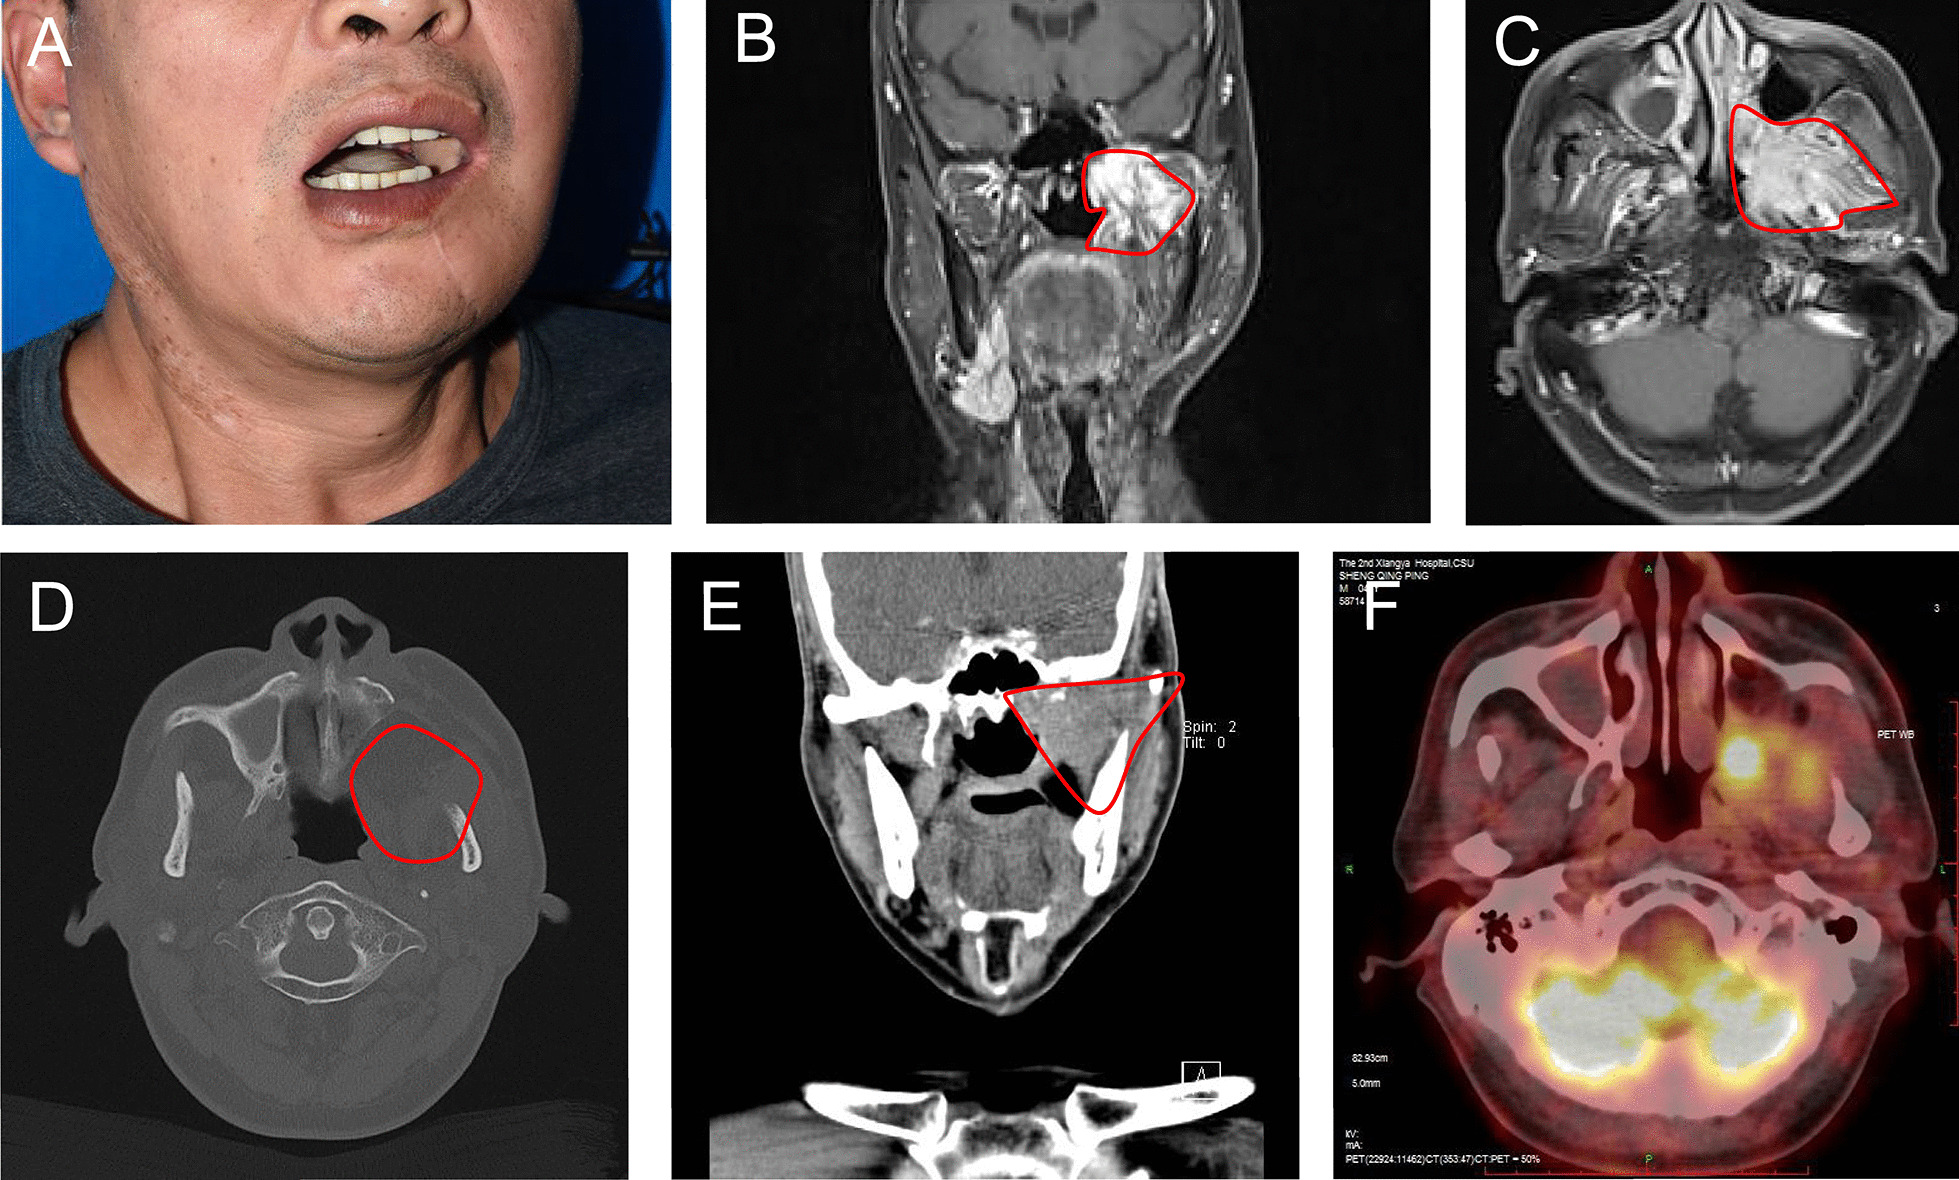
The recurrent POSCC located at basicranial region A The photograph of recurrent POSCC patient, B, C MR indicated that the recurrent tumor involved POAC, D, E CT indicated that bone destruction was demonstrated in mandibular ramus, infratemporal surface of maxillary body and pterygoid process, F PET-CT suggested the recurrent tumor located at basicranial region
